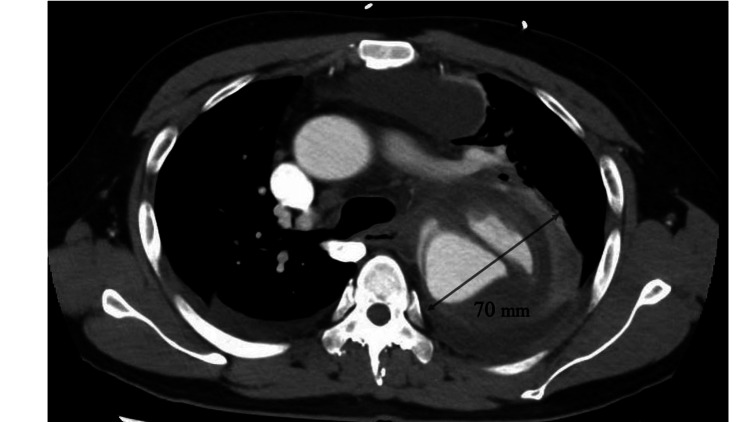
Initial imaging study of the chest Contrast-enhanced chest CT finding showing a 70-mm aneurysm in the distal aortic arch with dissection extending from the distal aortic arch to the common iliac artery. CT, computed tomography
